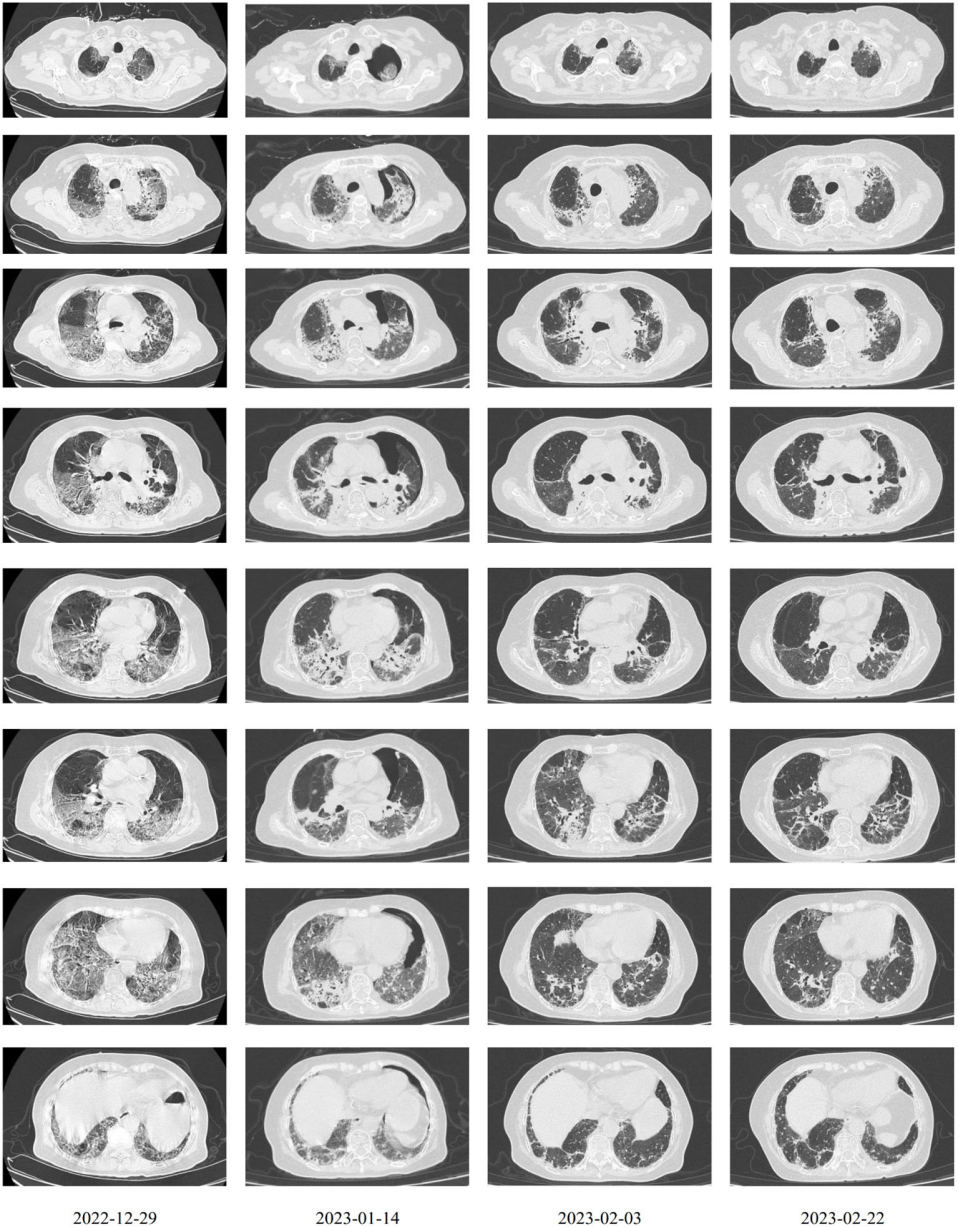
Characteristics of CT graphs.
